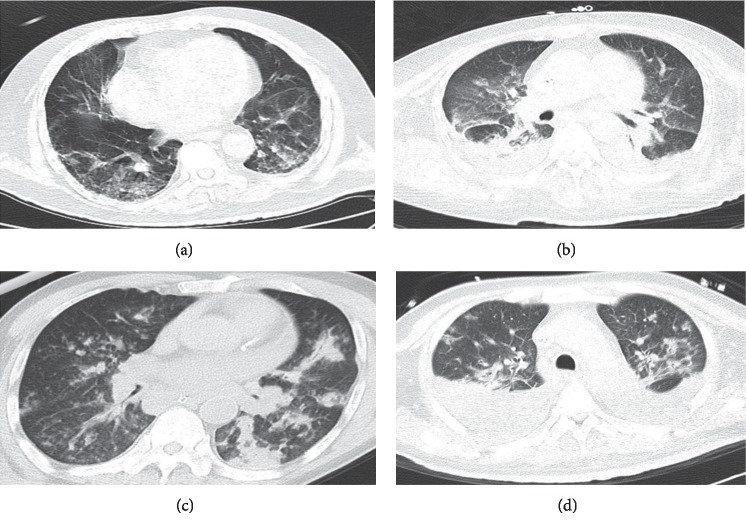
Chest CT findings in patients with IBV-related pneumonia. (a) 74-year-old man. Initial chest CT scan (day 10 after the onset of illness) shows bilateral diffuse interstitial infiltrates. (b) 64-year-old woman. Chest CT scan obtained on day 9 shows apparent consolidations accompanied by ground-glass opacities and pleural effusions. (c) 70-year-old man. Chest CT scan 9 days after the onset shows focal consolidations in the left lung. (d) 54-year-old man. Initial CT scan obtained on day 3 shows diffuse alveolar infiltrates with pleural effusion in both lungs.
